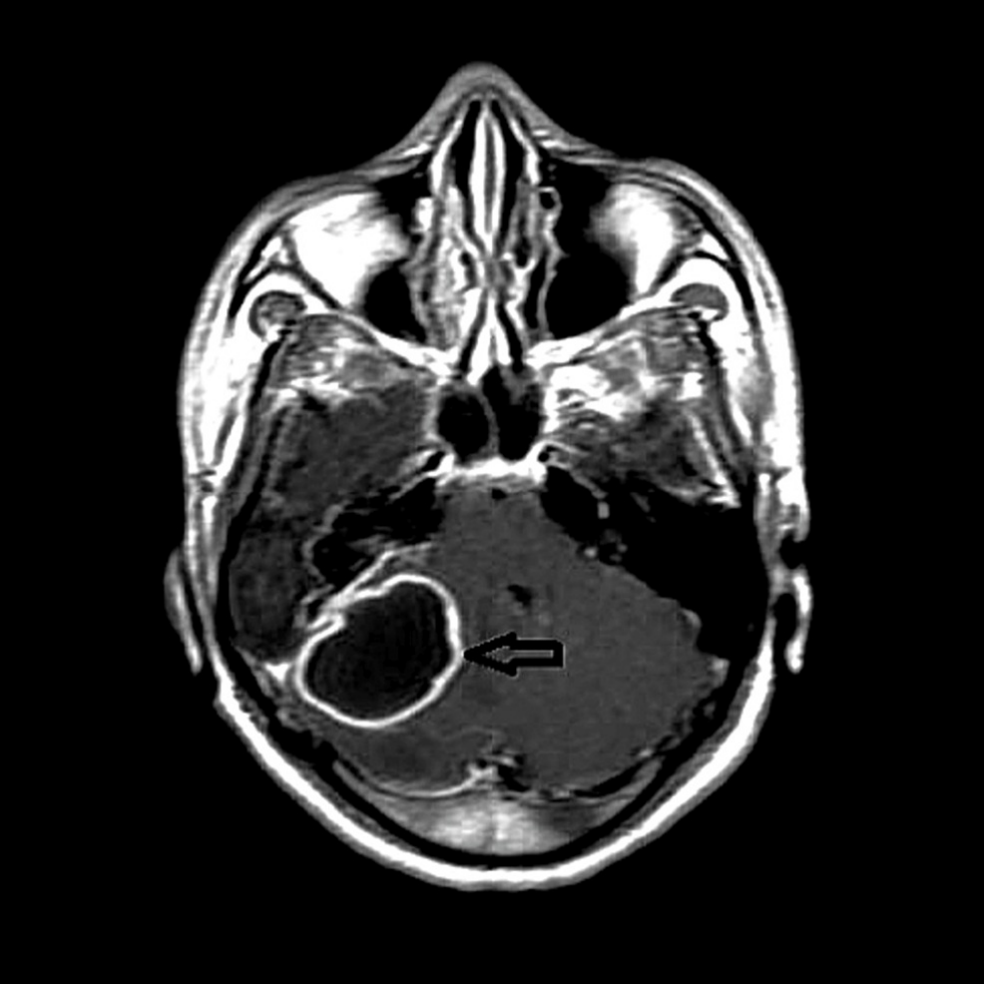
T2 weighted MRI brain (axial section) The image is showing a peripherally rim-enhancing altered signal intensity lesion in the right cerebellar hemisphere (arrow).
